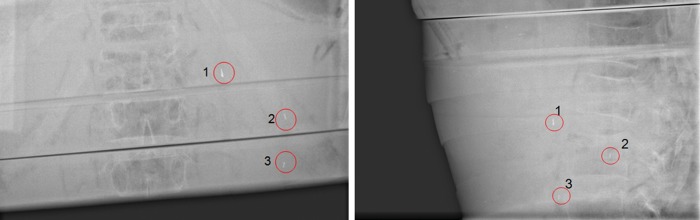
Orthogonal on-board kilovoltage imaging radiographs (OBI). Posterior-anterior (left) and lateral (right) view.
